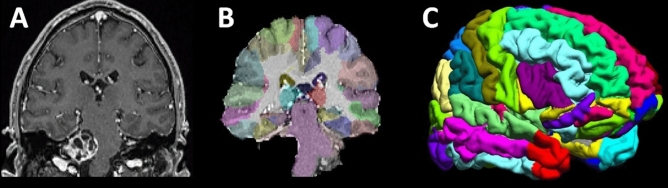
(A) Exemplary MR image of a patient with a T3 vestibular schwannoma of the right side. Data processing included skull striping and brain extraction process followed by the automated brain atlas registration (B,C).
